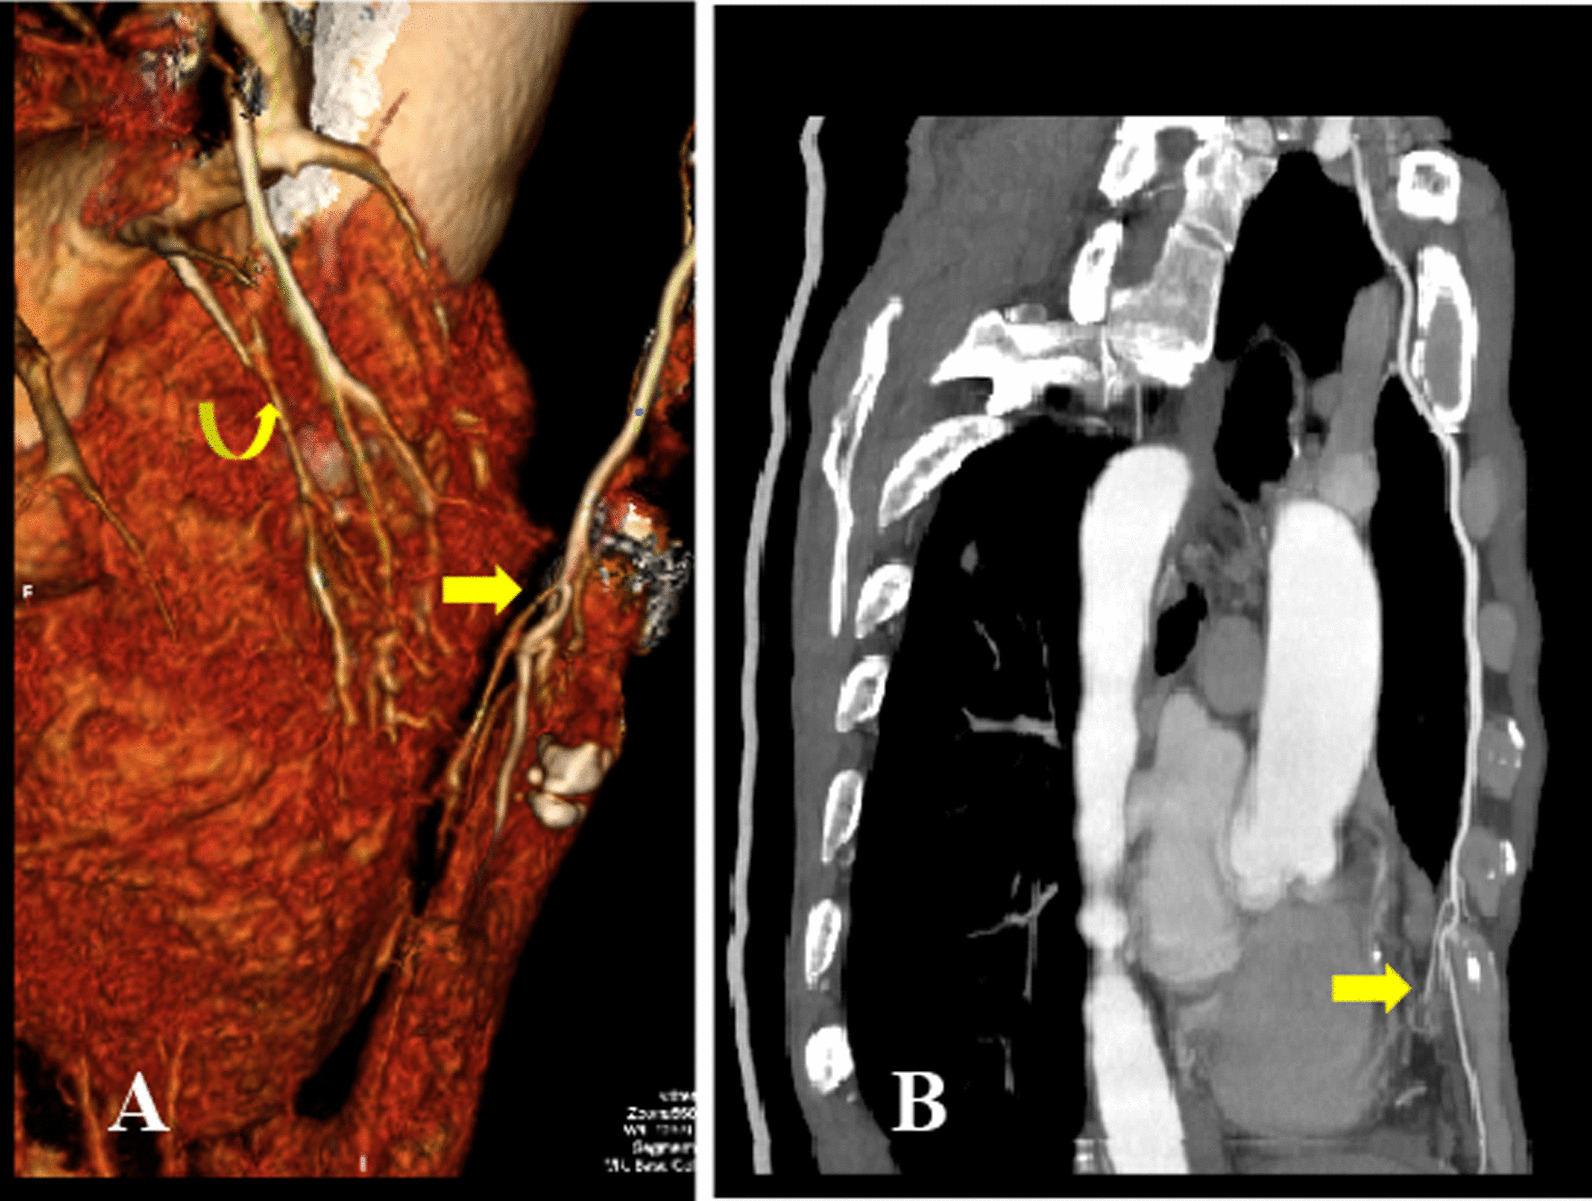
A 3D multidetector computed tomography volume rendering image shows an aberrant artery from the right internal mammary artery (straight arrow) and venous drainage (curved arrow) via the pulmonary veins to the right atrium. B Curved projection reformation (CPR) image shows the anomalous artery (straight arrow) arising from the internal mammary artery to the sequestered lung
